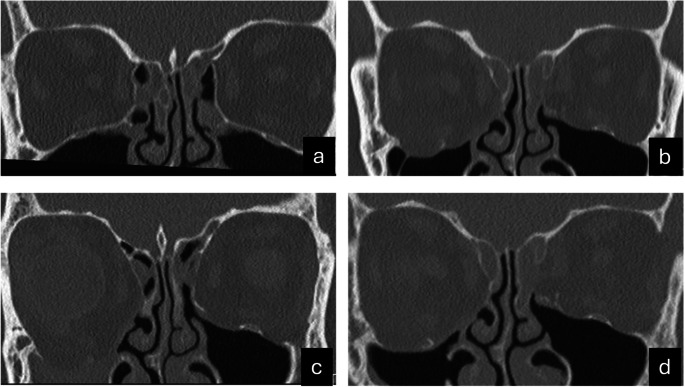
Iatrogenic secondary silent sinus syndrome (SSS). Note: Coronal CT images of a 51-year-old woman with Graves orbitopathy. (A). Before orbital decompression, the maxillary sinus shows no pathology. (B). After external orbital decompression of the right medial wall and orbital floor with involvement of the inferomedial strut (T1), adequate aeration of the right maxillary sinus is observed. Note the opacification of the ethmoid sinuses, most likely related to the decompression procedure. (C) Despite an uneventful postoperative course, progressive right enophthalmos and hypoglobus were noted, though the patient did not experience any symptoms of diplopia. The CT scan shows signs of secondary SSS (T2), including opacification of the right maxillary sinus, further depression of the orbital floor, and lateral deviation of the uncinate process. (D). Twelve months post-surgery, the CT scan shows a recovery of maxillary sinus aeration. Due to obstruction of the middle meatus caused by orbital prolapse, a Claoué procedure (inferior meatal antrostomy) was performed to restore function.
